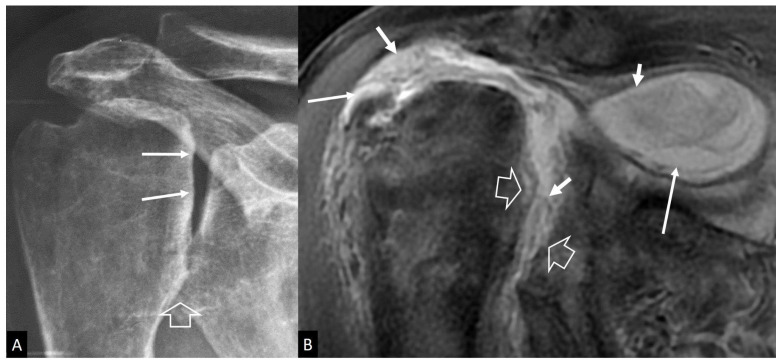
A 77-year-old male patient with mild pain, reduced range of motion, crepitation, and joint instability lasting 1 year prior to imaging. (A) Characteristic radiographic features of “Milwaukee” syndrome showing loss of the round configuration of the humeral head (arrows) and joint space narrowing at the inferior glenohumeral joint (open arrow). (B) Coronal oblique, fat-suppressed PD-w MR image showing massive rotator cuff tear, large joint effusion extending to bursae (long arrows), internal debris and synovial proliferation (short arrows), and subchondral bone and cartilage destruction (open arrows).
